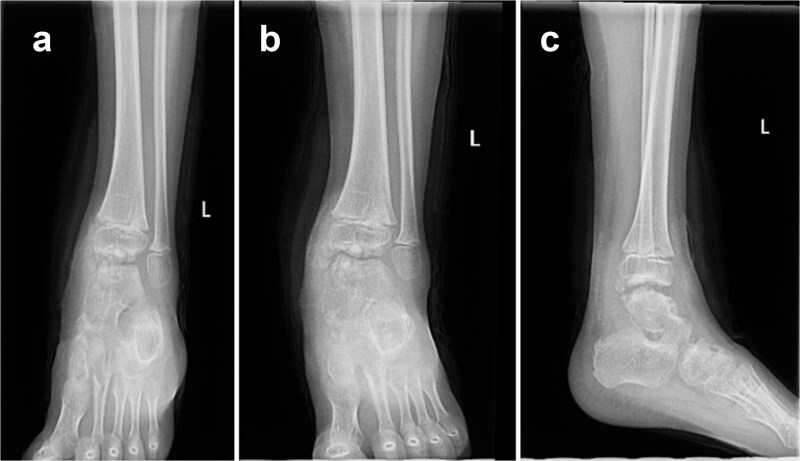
Preoperative AP (a), mortise (b), and lateral (c) ankle radiographs obtained prior to the second surgery demonstrating osteochondromas and irregularity involving the talar dome and distal tibia.
